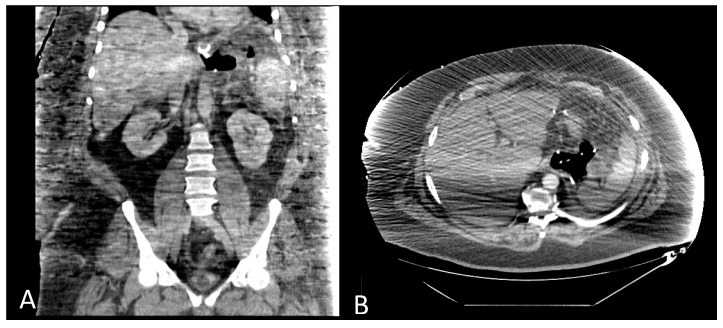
(A,B) Coronary and transversal slides of a CT scan showing two double-pigtail stents placed into an abdominal cavity between the stomach and spleen.
